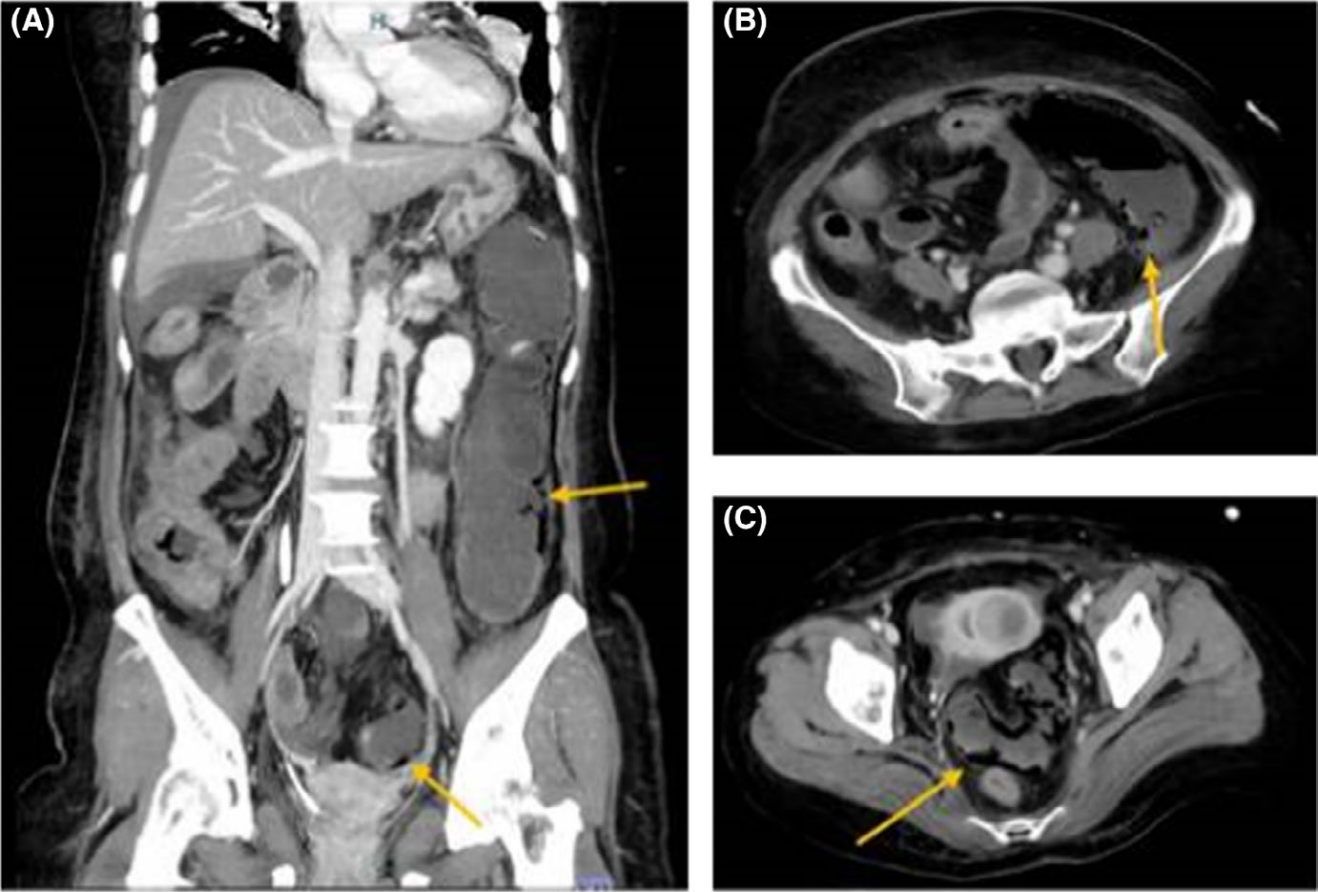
Abdominal CT on the 4th day. (A) Sagittal image. (B, C) Coronal section. Pneumatosis intestinalis is observed in the descending and sigmoid colon
